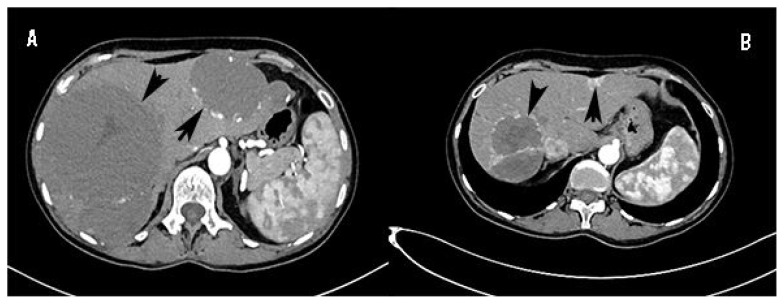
A: Arterial phase CT image of a hepatic giant hemangioma before TACE treantment. Arrows show the lesions. B: Arterial phase CT image of a hepatic giant hemangioma 12 months after TACE treatment. Arrows show the lesions.
